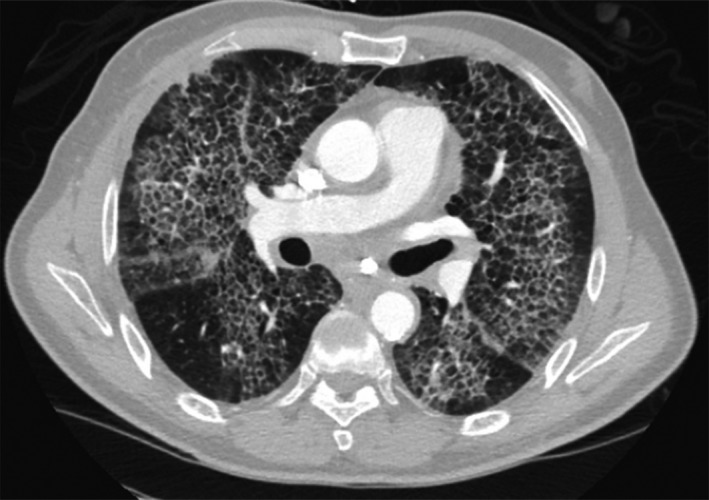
CT scan showing characteristic “crazy paving pattern” with polygonal honeycomb‐like interlobular septal thickening.
